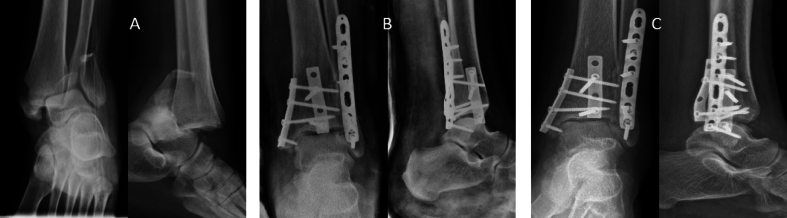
A case of 44B3 ankle fracture with an AO at the end of follow-up. A: pre-operative x-rays. B: after surgery x-rays. C: seven years follow-up x-rays. Last images show a reduction of joint space and sclerosis of the subchondral bone.
